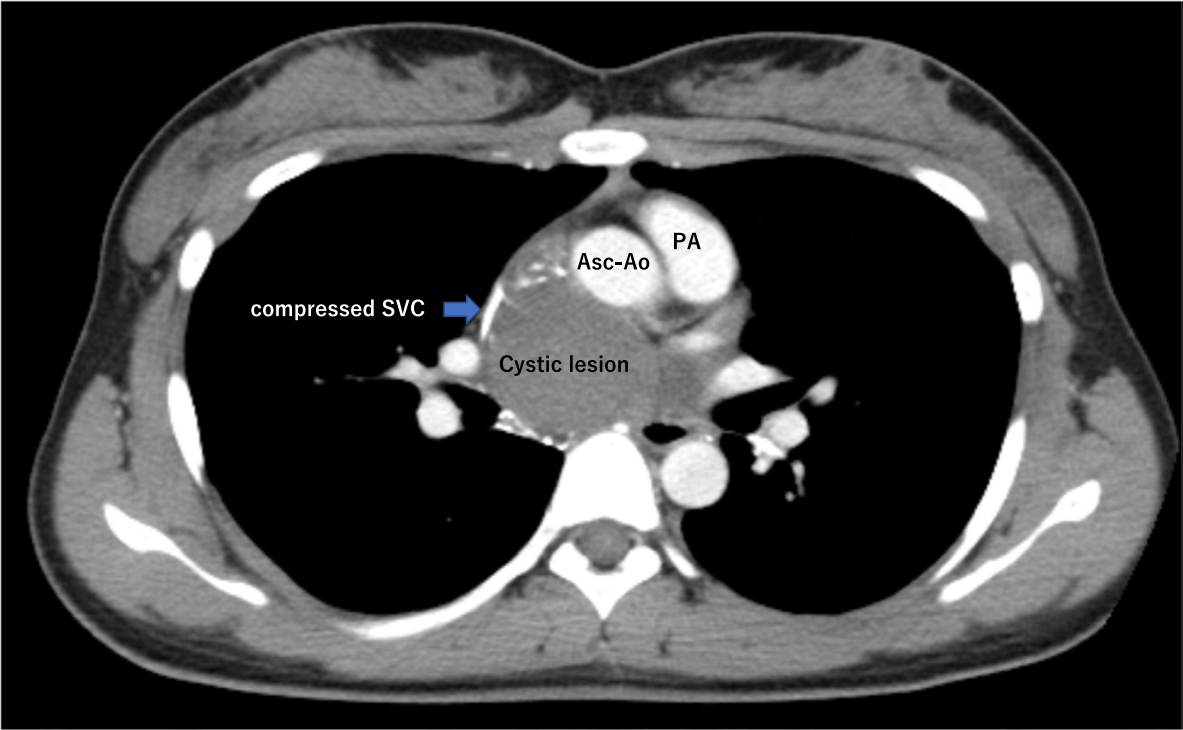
Preoperative CT scan indicating the compressed SVC
